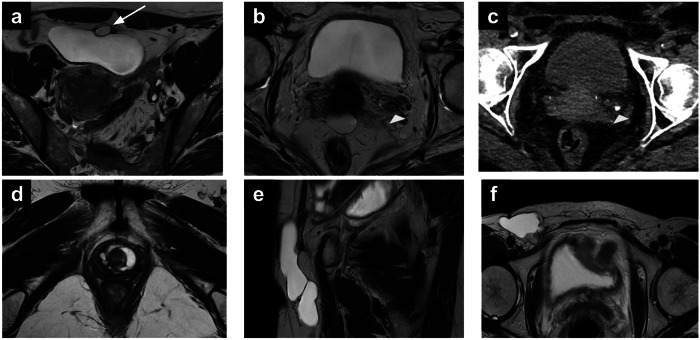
Urachal cyst (a). Axial T2-WI (a) showing a urachal cyst (arrow) in a 43-year-old patient undergoing pelvic MRI for endometriosis. Ureteric calculus (b, c). Axial T2-WI (b) of a 69-year-old patient with recurrent vulvar cancer showing a filling defect in the left distal ureter (arrowhead), with associated ureteric wall thickening, then confirmed as a calcified calculus at subsequent CT. UD (d). Axial T2-WI (d) of a 53-year-old patient with locally advanced cervical cancer showing a multicystic lesion with fluid content surrounding the urethra, in keeping with a UD. Compression of the anterior vaginal wall can be noted. Nuck canal hydrocele (e, f). Sagittal T2-WI (e) and axial T2-WI (f) of a 54-year-old patient with vaginal cancer; an elongated cystic lesion with fluid content and thin walls can be noted in the right inguinal region (canal of Nuck), representing a Nuck canal hydrocele. WI, weighted images
